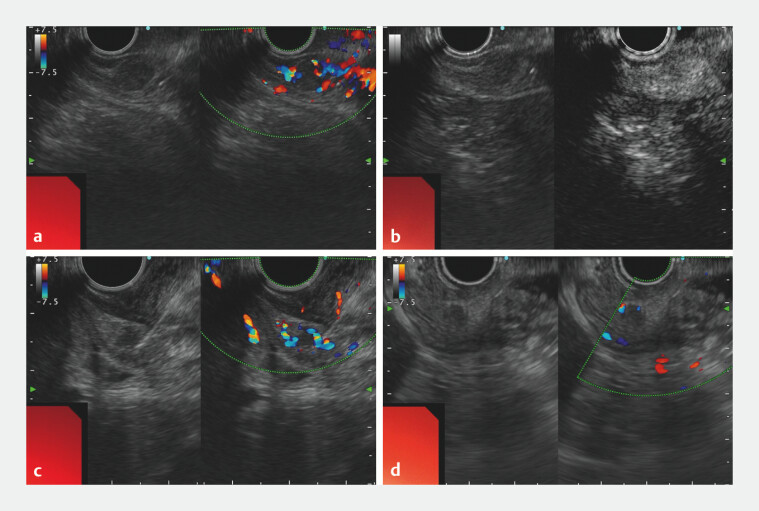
Endoscopic ultrasound (EUS)-guided lauromacrogol embolization for suspected gastrinomas in the gastric antrum.
